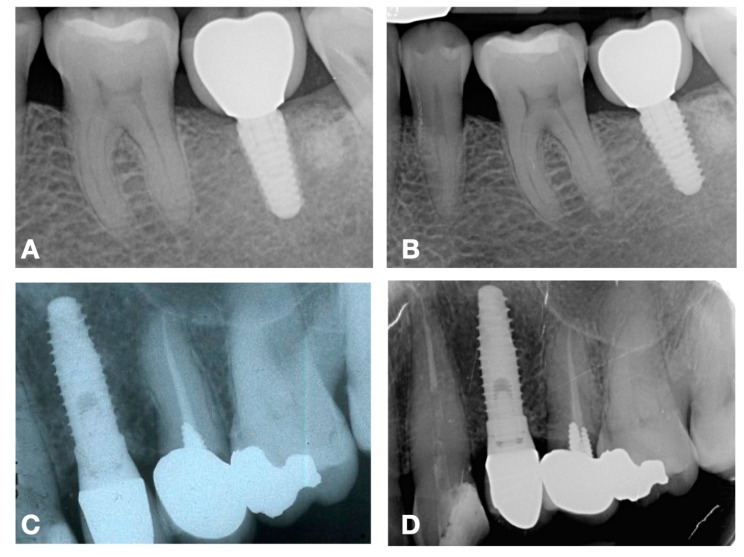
Radiographic evaluation of two different implant-prosthetic cases at baseline (A,C) and at 4 (B) and 8 (D) years of follow-up.
